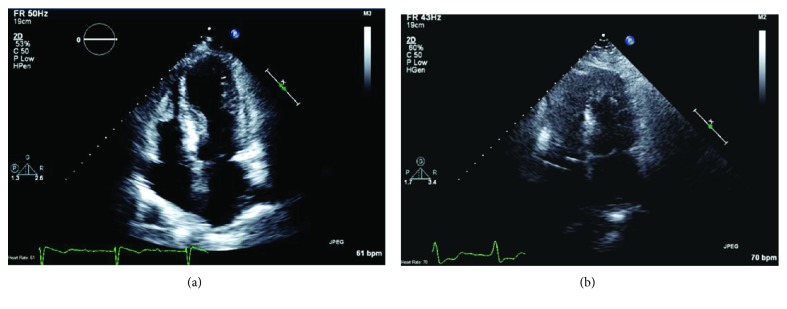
Apical 4 chamber ECHO view (a) on presentation suggestive of takotsubo cardiomyopathy followed by repeat apical 4 chamber view (b) two months later showing recovery of previously noted wall motion abnormalities.
